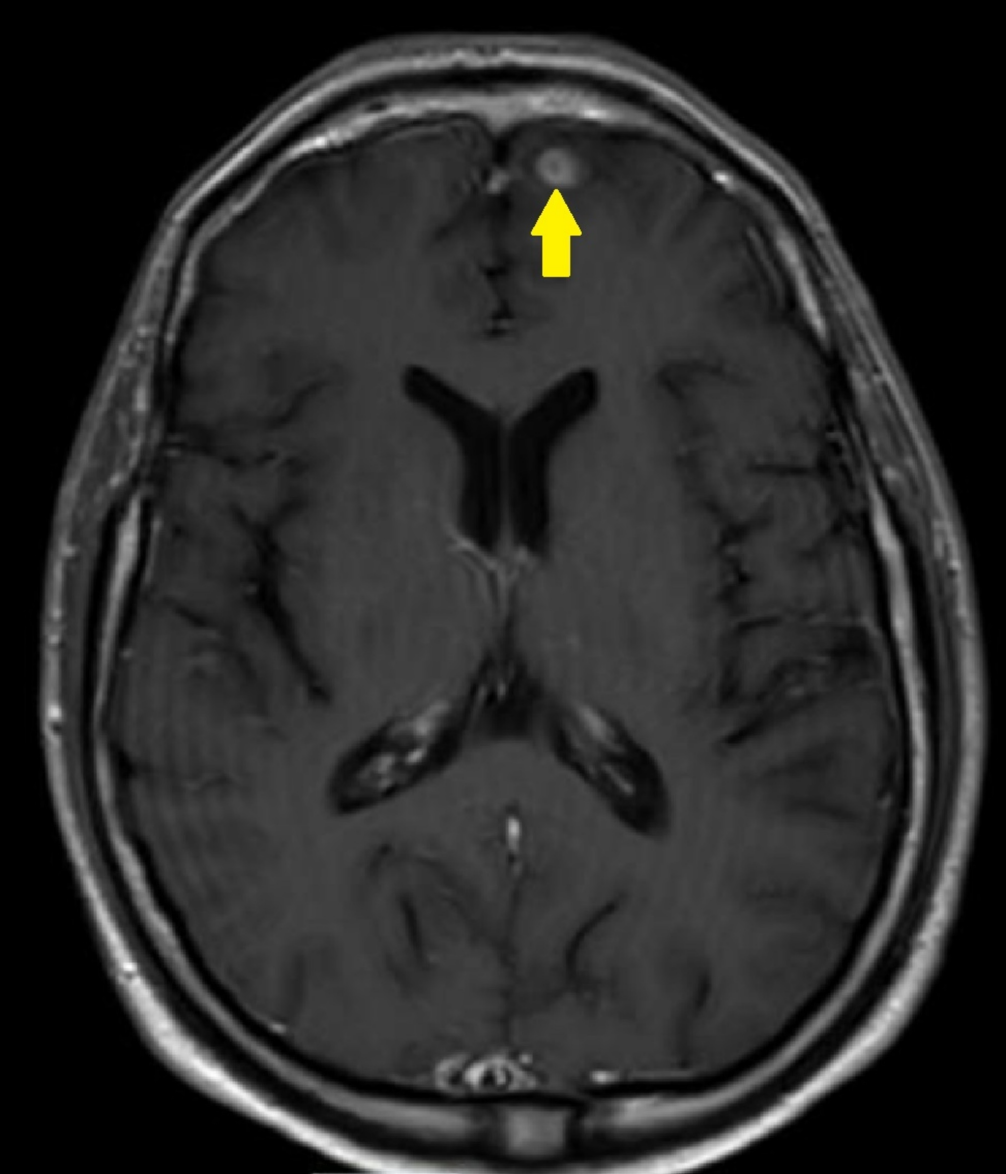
Left Frontal Lobe Metastasis Pre-Treatment Transverse Brain Magnetic Resonance Imaging (MRI). Small 6 mm ring-enhancing metastatic lesion noted in left frontal lobe with some surrounding edematous change.
